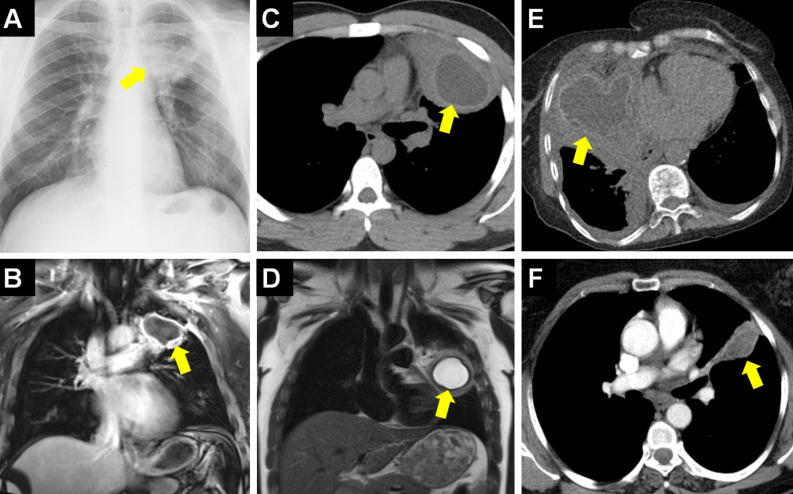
Chest radiograph (A) shows opacity (arrow) belonging to a ruptured and complicated hydatid cyst (HC), and post-contrast magnetic resonance imaging (MRI) (B) of the same patient shows the “ring enhancement sign” (arrow) indicating peripheral contrast enhancement of the cyst wall. The HC is seen as low density on axial computed tomography (CT) (C) and the “hypointense rim sign” in images of the same patient with a hypointense wall on T2-weighted MRI (D) (arrows). Axial CT (E) shows the collapsed endocyst layer (arrow) within the complicated lung parenchyma of a ruptured HC, and in another patient (F), an HC causing atelectasis in the surrounding lung parenchyma (arrow) is seen.
